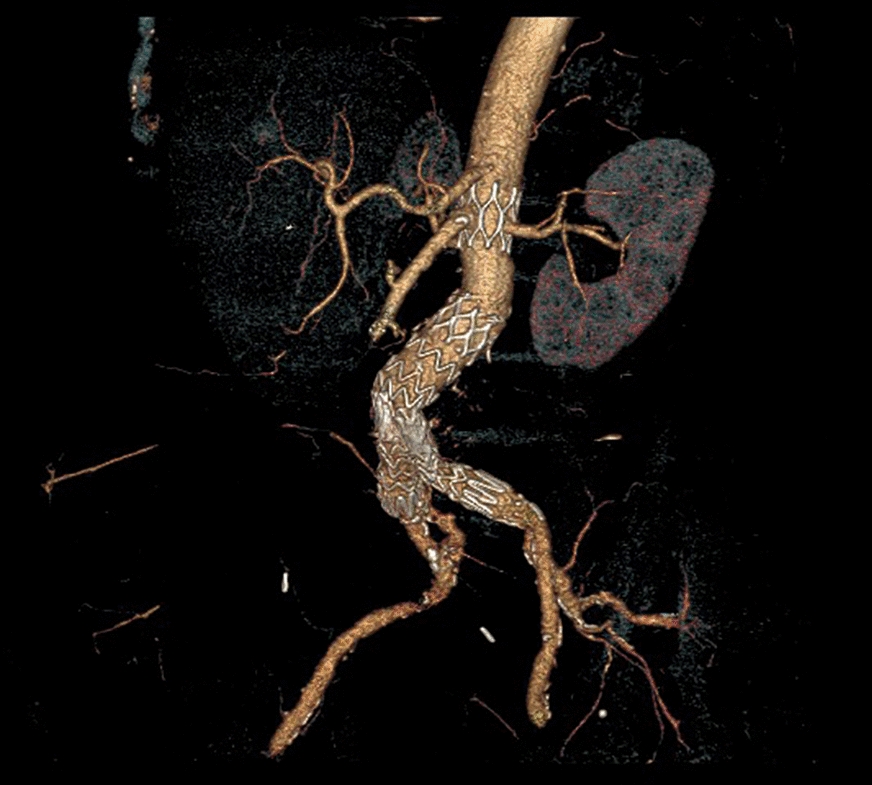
24-month CT-scan showing disconnection of the bare suprarenal stent from main body stent-graft
